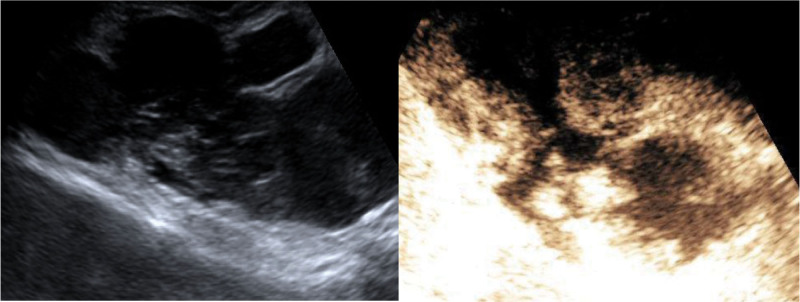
CEUS of the mass: 17 seconds after the injection of contrast agent evaluated by the convex probe. CEUS showed a high-enhancement centripetal area, uneven internal enhancement, visible enhanced bridge, and non-enhanced central area. CEUS = contrast-enhanced ultrasound.
